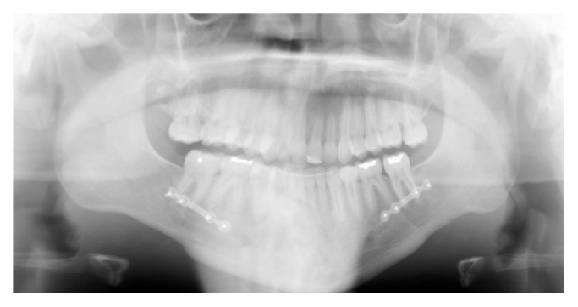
Stage 2: Orthodontic Treatment Final Panoramic Radiograph.
